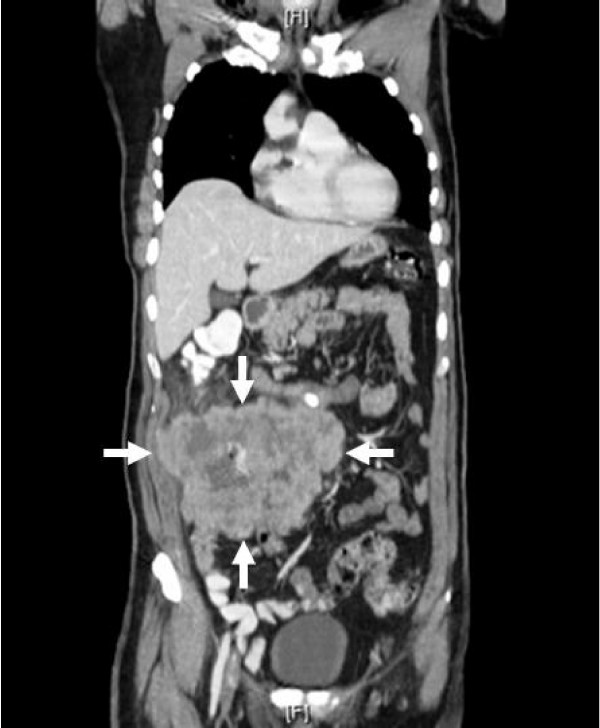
Frontal view of Computed Tomography (CT), which revealed a large 13 × 12 × 16 cm right-sided ill-defined soft tissue mass involving the ascending colon.
